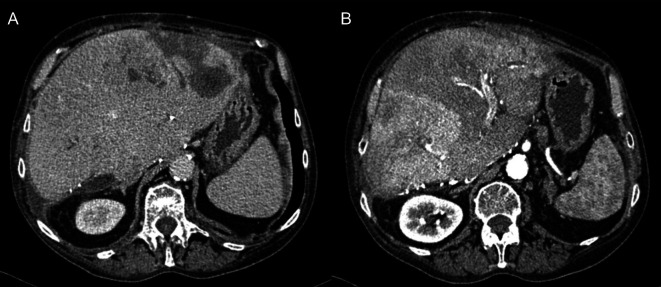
Abdominal CT scan after 12 months in venous (A) and arterial phase (B) showing slight progression of multicentric HCC nodules with regression of hematomas and abscesses.
